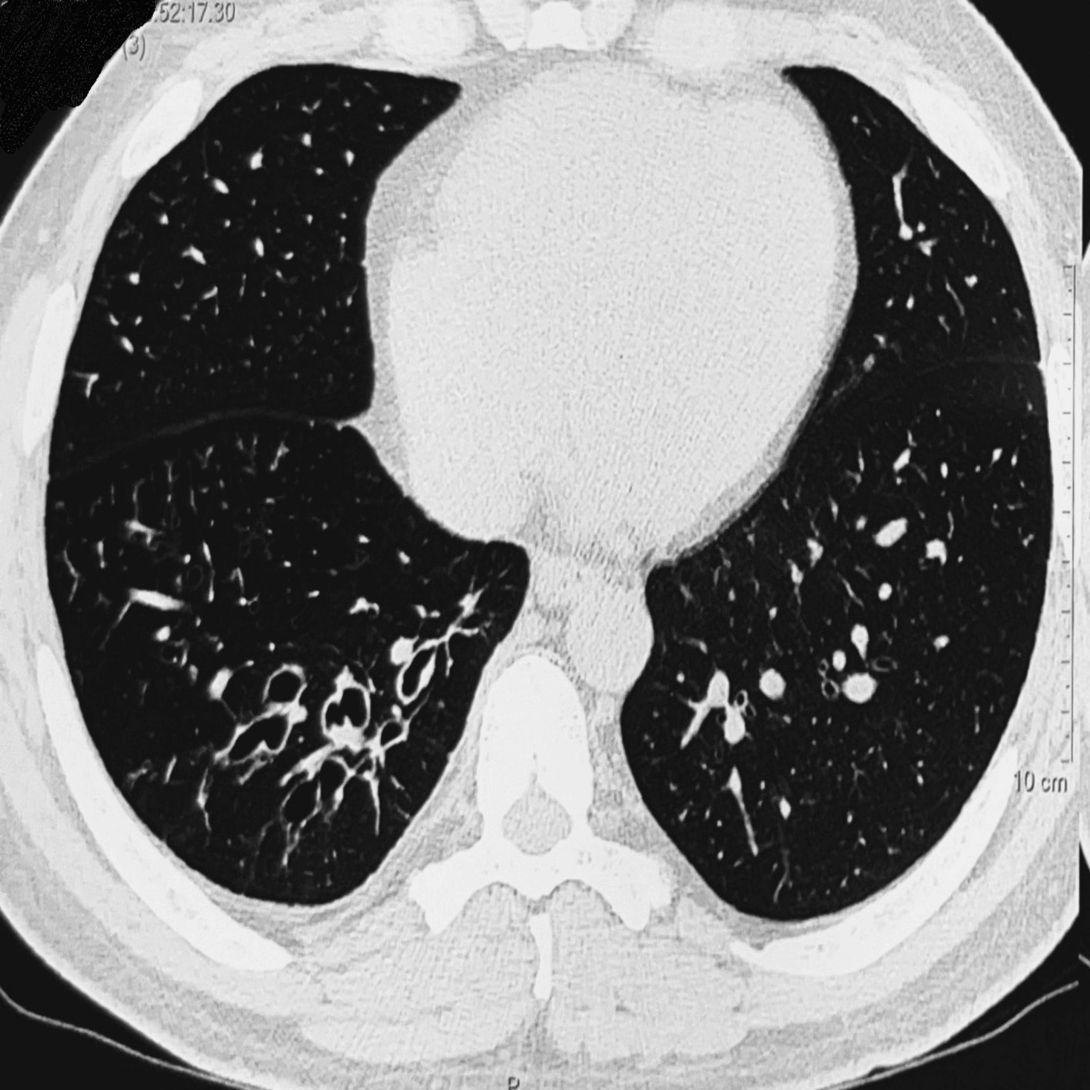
Lung window of follow-up CT scan three months after foreign body retrieval showing bronchiectasis in the right lower lobe.
